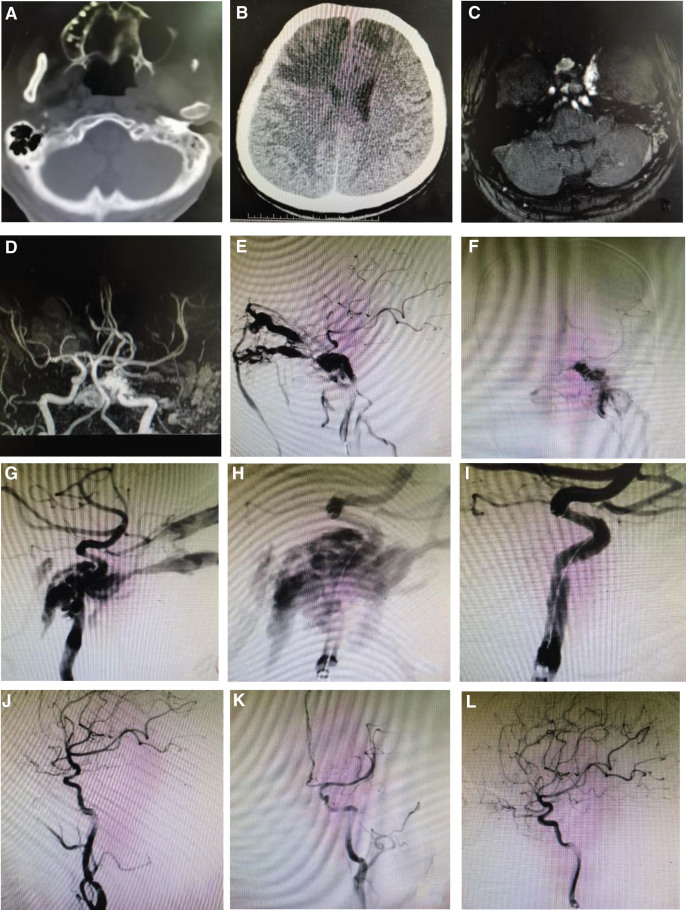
(A) Head CT shows nasal bone and occipital bone fracture. (B) Brain CT showed frontal lobe contusion and laceration. (C) Abnormal high signal intensity of cavernous sinus on MRI. (D) MRA showed more tortuous vascular shadows at the siphon of the left internal carotid artery. (E‐G) DSA showed that the left internal carotid artery cavernous sinus segment had a large amount of contrast agent infiltrating into the cavernous sinus, and the superior, inferior and peripheral venous vessels were significantly dilated. (H) Intraoperative angiography after placement of covered stent. (I‐K) Immediately after the release of the covered stent, angiography, lateral and anteroposterior angiography showed that the fistula was completely covered, and the original vein vascular mass was developed and disappeared. (L) Six months after operation, DSA showed that the fistula disappeared completely, did not recur, and the blood flow in the stent was smooth. CT = computed tomography, DSA = digital subtraction angiography, MRA = magnetic resonance arteriography, MRI = magnetic resonance imaging.
